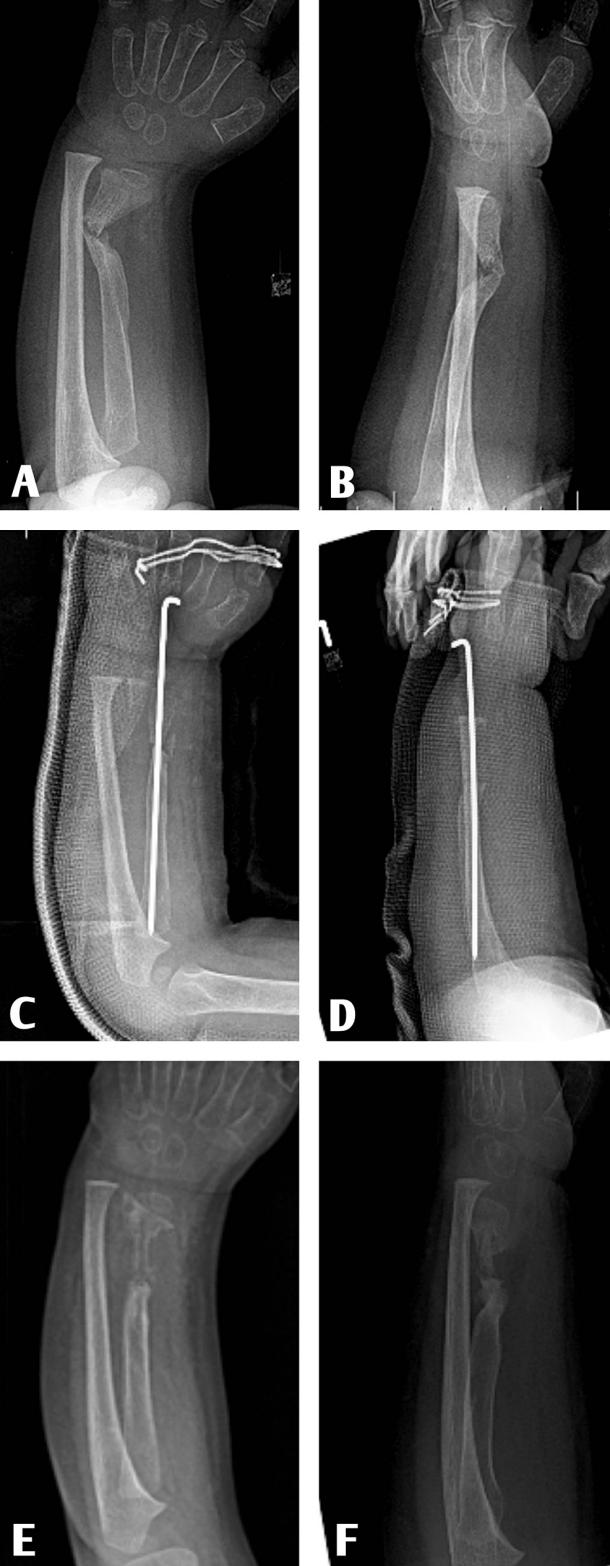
Interventions performed on the patient at two years of age. A, B. X-ray after cast immobilization. C, D. X-ray after internal fixation + grafting. E, F. X-ray after electrical stimulation for one month.
